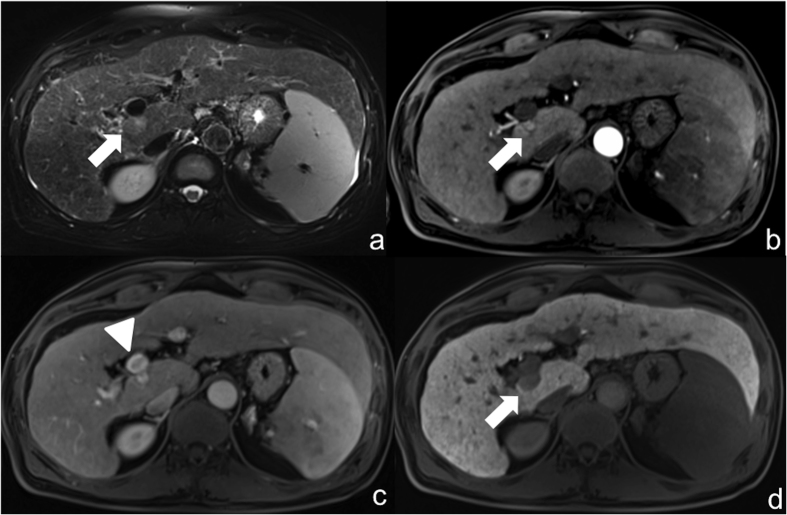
Same patient as in Fig. 1: pre-interventional MRI with liver-specific contrast agent (Gd-EOB-DTPA): (a) Hyperintense visualization of the HCC (arrow) in native fat-saturated T2-weighted sequence posterior to the main stem of the portal vein at the transition to liver segment I. (b) Dynamic T1-weighted fat-saturated sequence after contrast in arterial phase shows sluggish arterial hypervascularization of the HCC (arrow).(c) Dynamic T1-weighted fat-saturated sequence after contrast in portal venous phase shows the directly adjacent main stem of the portal vein (tip of arrow). (d) T1-weighted fat-saturated sequence in hepatobiliary phase with wash-out (arrow).
